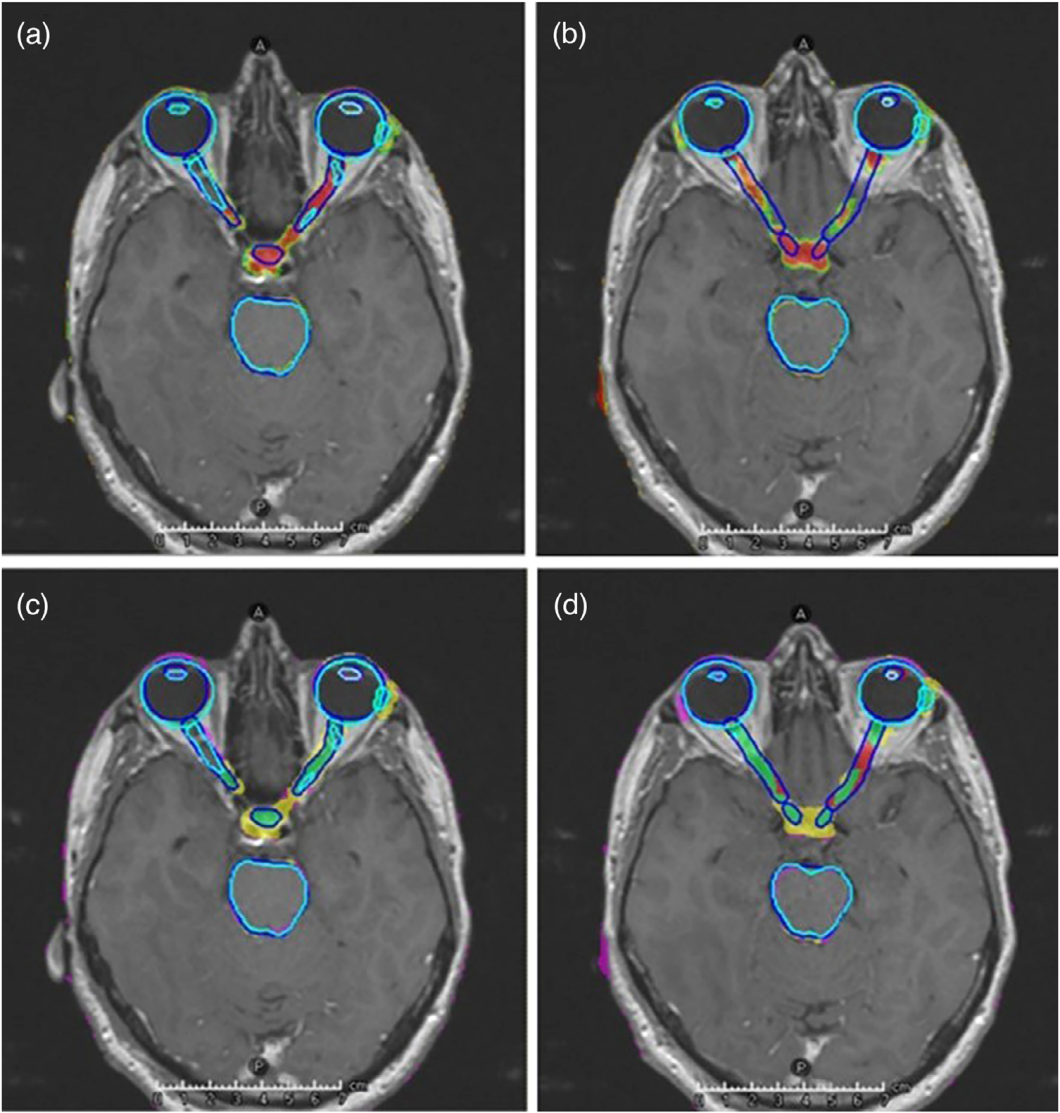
Axial T1w‐Gd MRI with dark‐blue contours representing gold standard and light‐blue representing EM‐LQ autosegmentation. (a) Example showing a high uncertainty level for missing pituitary segmentation and errors in optic nerves. (b) High uncertainty for missing optic chiasm and apparent false‐negative predictions for optic‐nerves, which are in fact due to non‐anatomical GS contours (see main text). FP in the region near lacrimal glands is typical of ACo on MRI, where lacrimal glands are very hard to visualize and therefore highly uncertain in location. (c) and (d) Four‐color‐map showing regions of TP (green), FP (pink), and GDC modified FP (yellow) relative to the differences‐to‐gold‐standard, for the ACo prediction.
